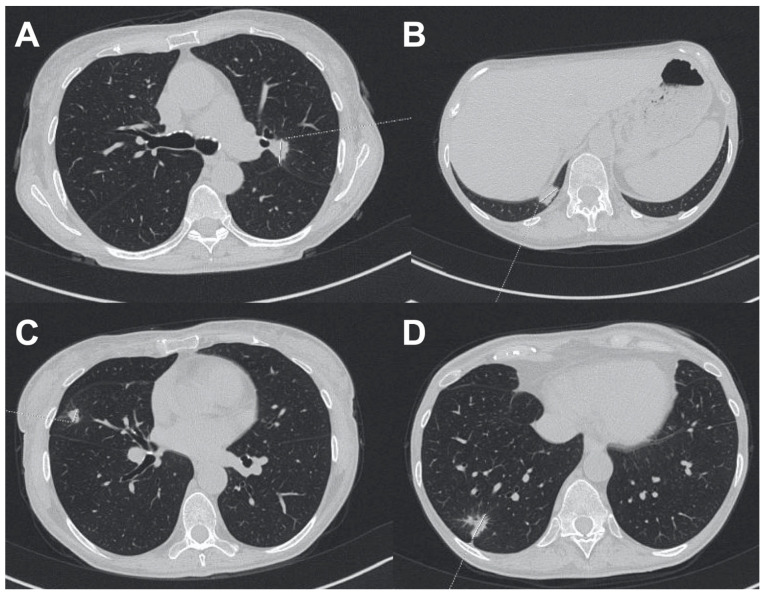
(A–D). CT of patient 2, with multiple pulmonary nodules found in the right and left lungs.
